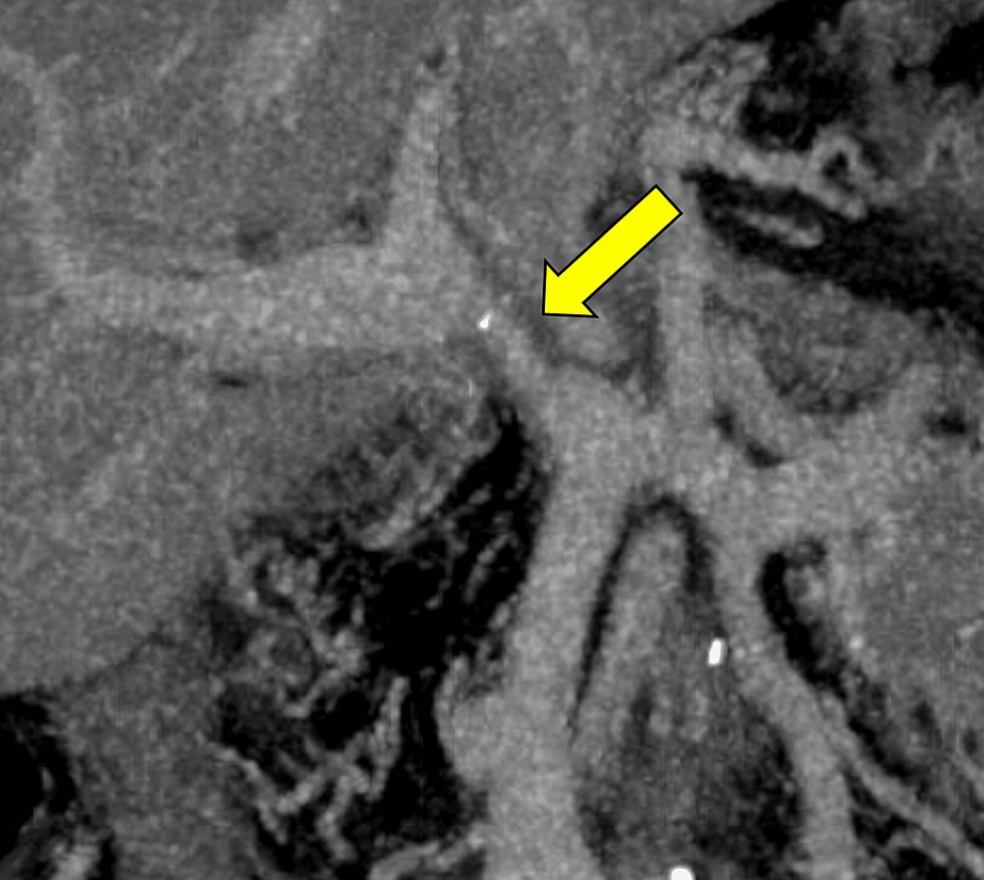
CECT reformatted coronal section. Stenosis of the extrahepatic portal vein before dilation (yellow arrow). CECT: Contrast-enhanced computed tomography.
